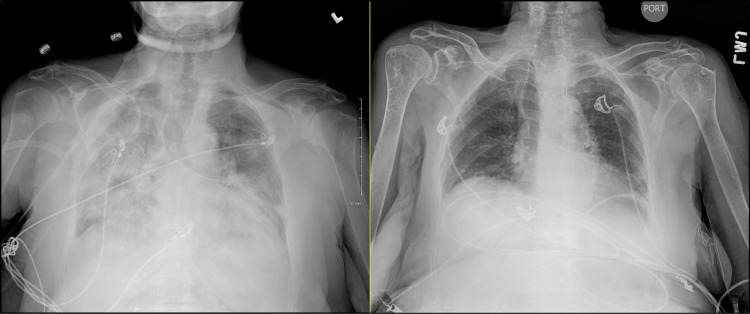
Chest X-ray (CXR) at admission (left) and a prior CXR for comparison (right). Diffuse bilateral pulmonary consolidation.
